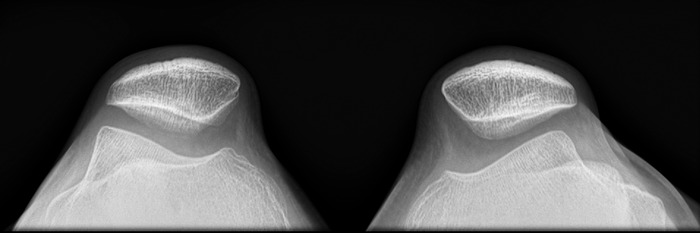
Merchant view radiograph shows left greater than right lateral patellar subluxation at 45° flexion.
